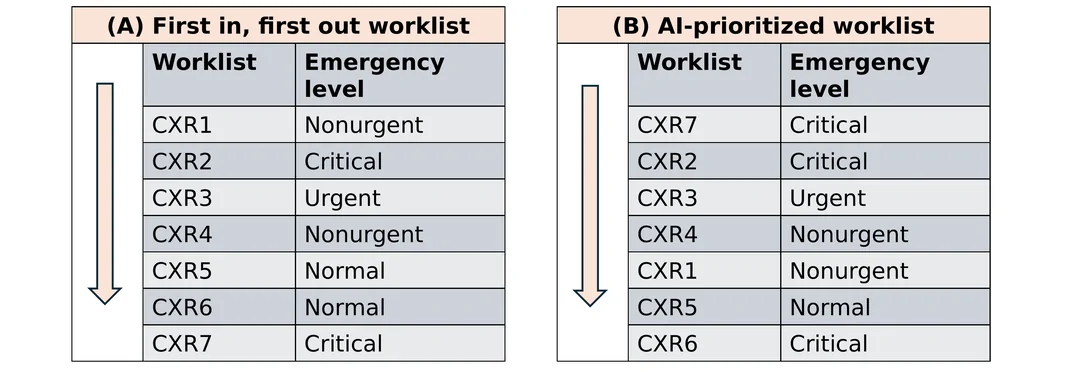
Comparison of worklist prioritization methods. (A) First-in, first-out worklist in session 1 (unassisted) vs (B) AI-prioritized worklist in session 2 (AI-assisted). CXR: chest radiograph.
